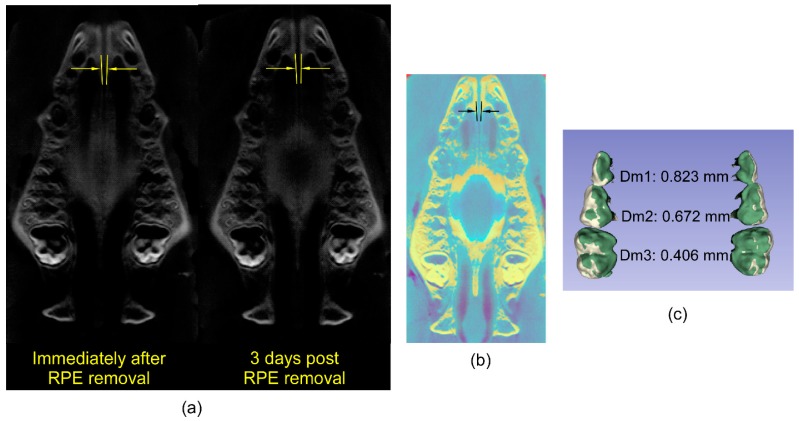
(a) Axial slices of CBCT images obtained immediately after RPE removal (left) and after three days of relapse (right) showing sutural separation demarcated by lines and yellow arrows). (b) Generalized superimposition of the palate immediately after RPE removal (cyan) and after three days of relapse (yellow) showing minimal changes in midpalatal suture separation. (c) Generalized superimposition of the maxillary dentition immediately after RPE removal (white) and after three days of relapse (green) showing the magnitude of relapse at the first (Dm1); second (Dm2) and third (Dm3) molars.
